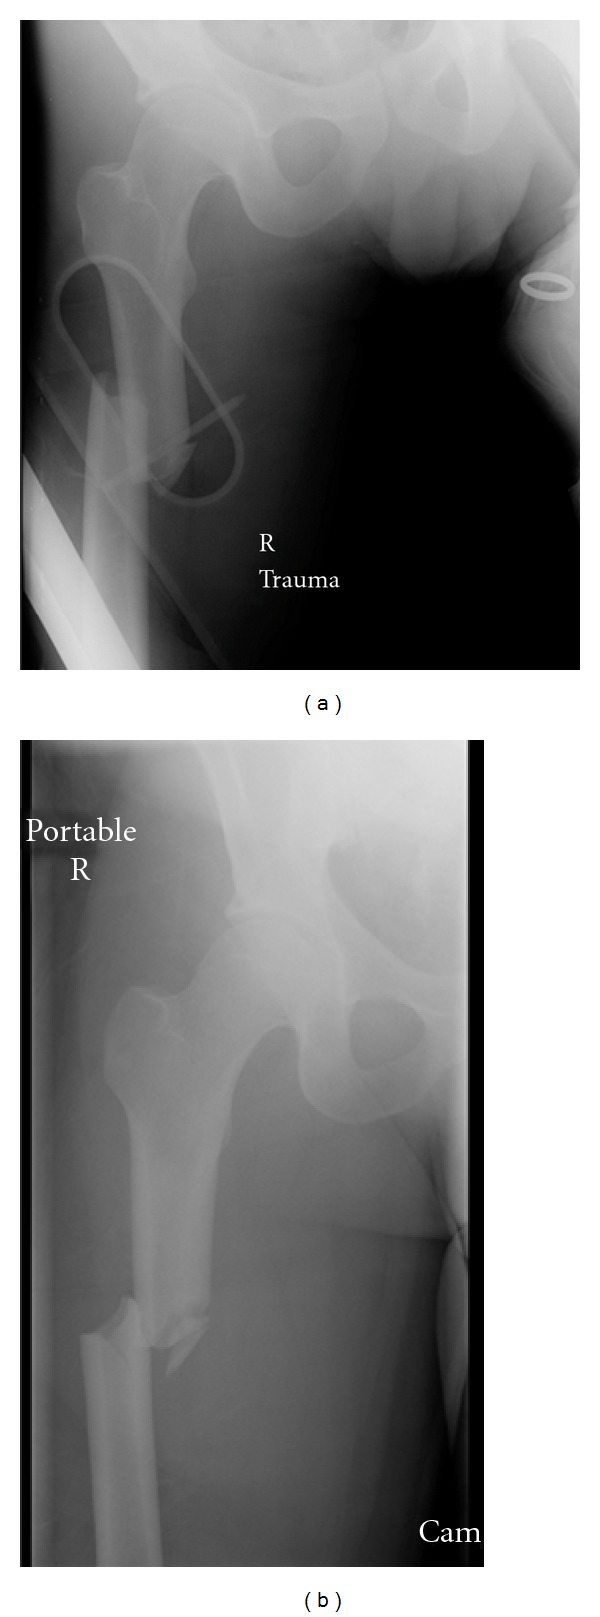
(a) Anteroposterior radiograph of closed right proximal femoral fracture, (b) anteroposterior radiograph of fracture with distal traction applied.
